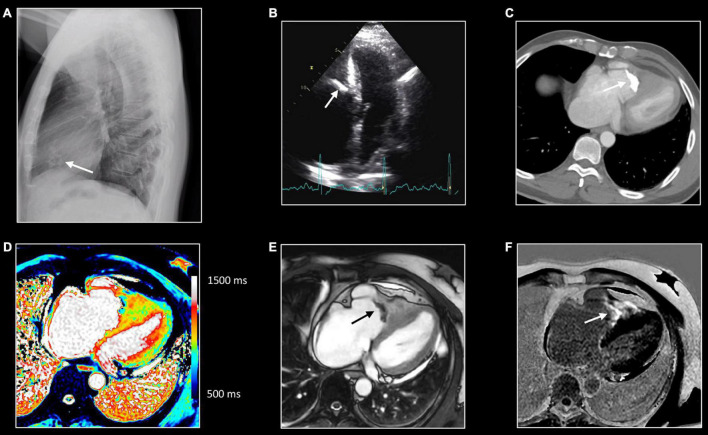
Right sided endomyocardial fibrosis. Sixty-seven-year-old man with extensive fibro-calcification of the right ventricular apex, severe tricuspid regurgitation, and dilatation of the right atrium, caval and hepatic veins. Lateral chest x-ray (A) shows amorphous calcifications (arrow) in the anterior half of the cardiac silhouette, which is confirmed at transthoracic echocardiography [arrow, (B)] as well on computed tomography (CT) [arrow, (C)]. Native T1 mapping (D), post contrast bSSFP cine imaging (E) and LGE imaging (F). All CMR images were acquired in horizontal long-axis. The obliteration of the right ventricular cavity by the fibrocalcific process can be well appreciated at CT and CMR. Native T1 mapping shows inhomogeneous appearance with strong enhancement at LGE imaging, reflecting extensive replacement fibrosis [arrow, (F)].
